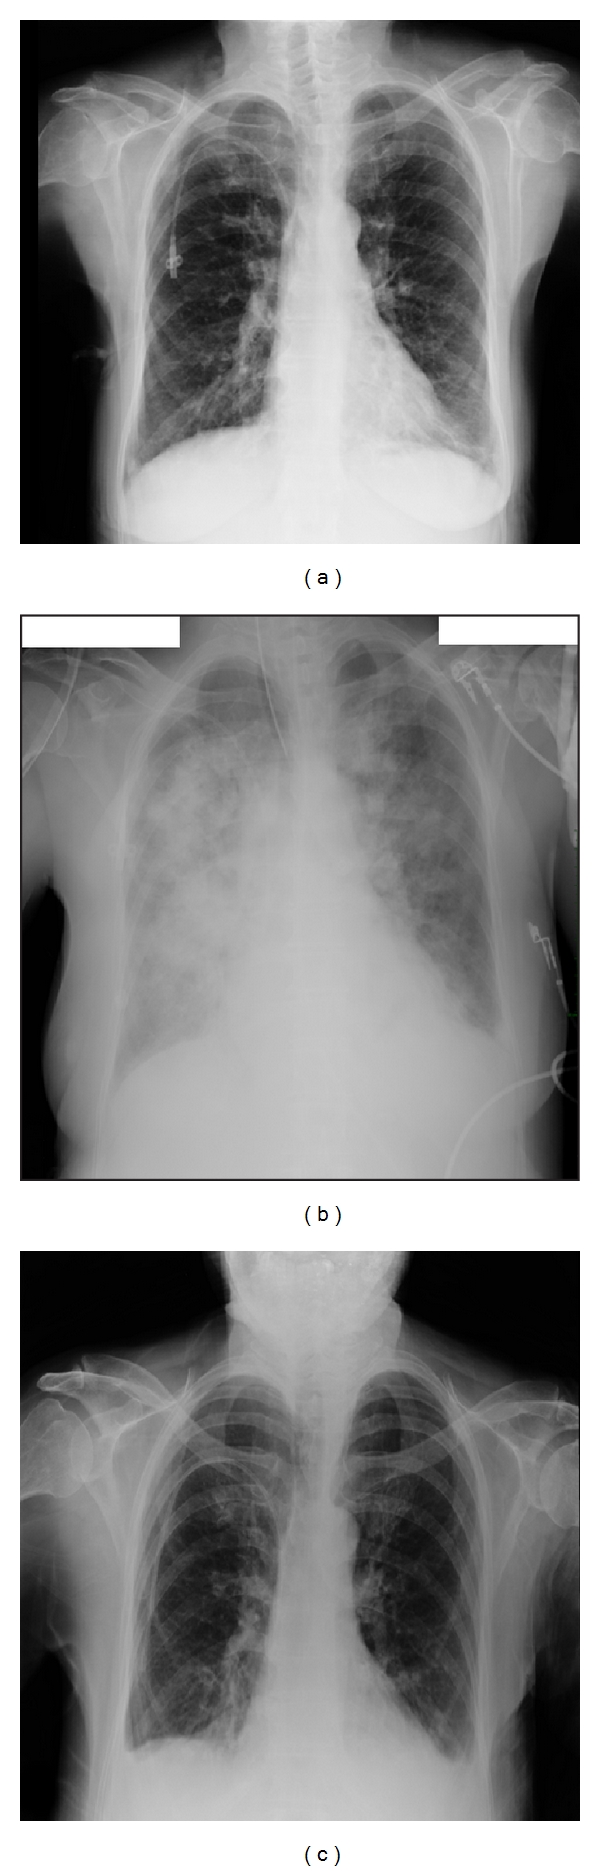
Chest radiographs of a patient with acute respiratory distress syndrome primary caused by influenza B virus infection. (a) On admission, no pulmonary infiltrates were found. (b) Chest radiograph shows bilateral diffuse alveolar opacities. (c) Chest radiograph a day prior to discharge shows complete resolution of the bilateral diffuse alveolar opacities.
